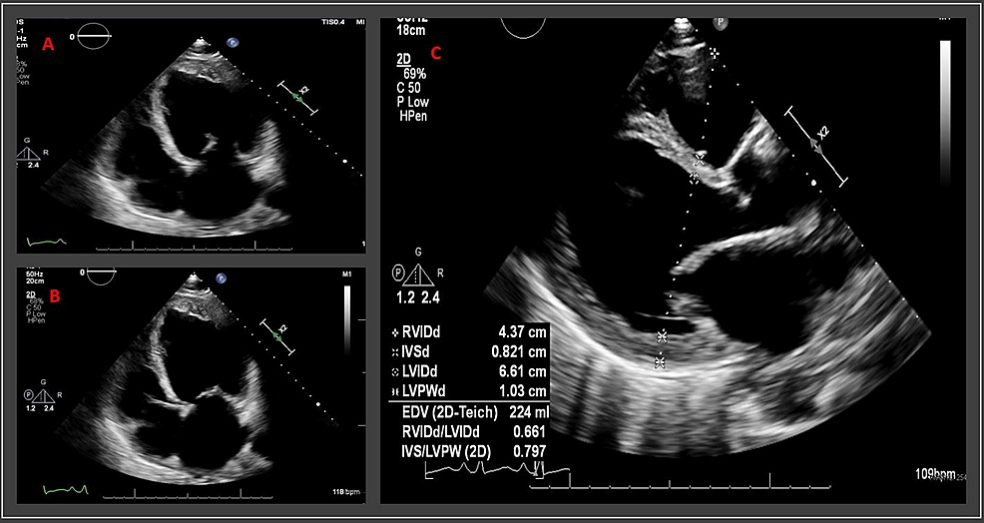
Transthoracic echocardiogram (TTE) Transthoracic echocardiogram (TTE) with apical four-chamber views in diastole (A) and systole (B) and parasternal long-axis view (C) demonstrated global left ventricular hypokinesis and markedly reduced left ventricular ejection fraction (EF) of 10-15%.
